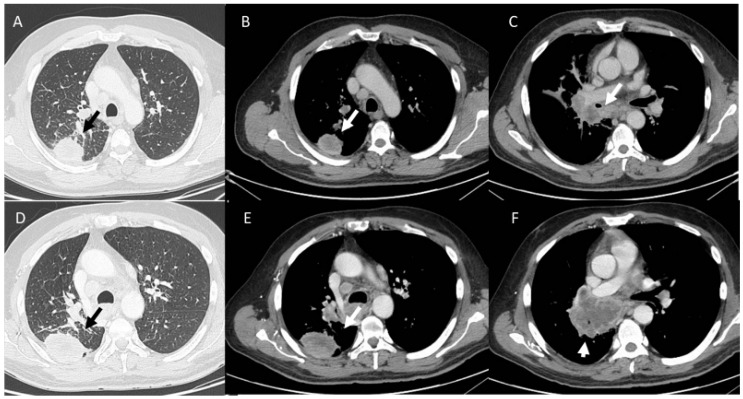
Iperprogression in an NSLC (arrows) patient treated with immunotherapy. CT assessment in pre-treatment phase (A–C) and after 3 months (D–F).
